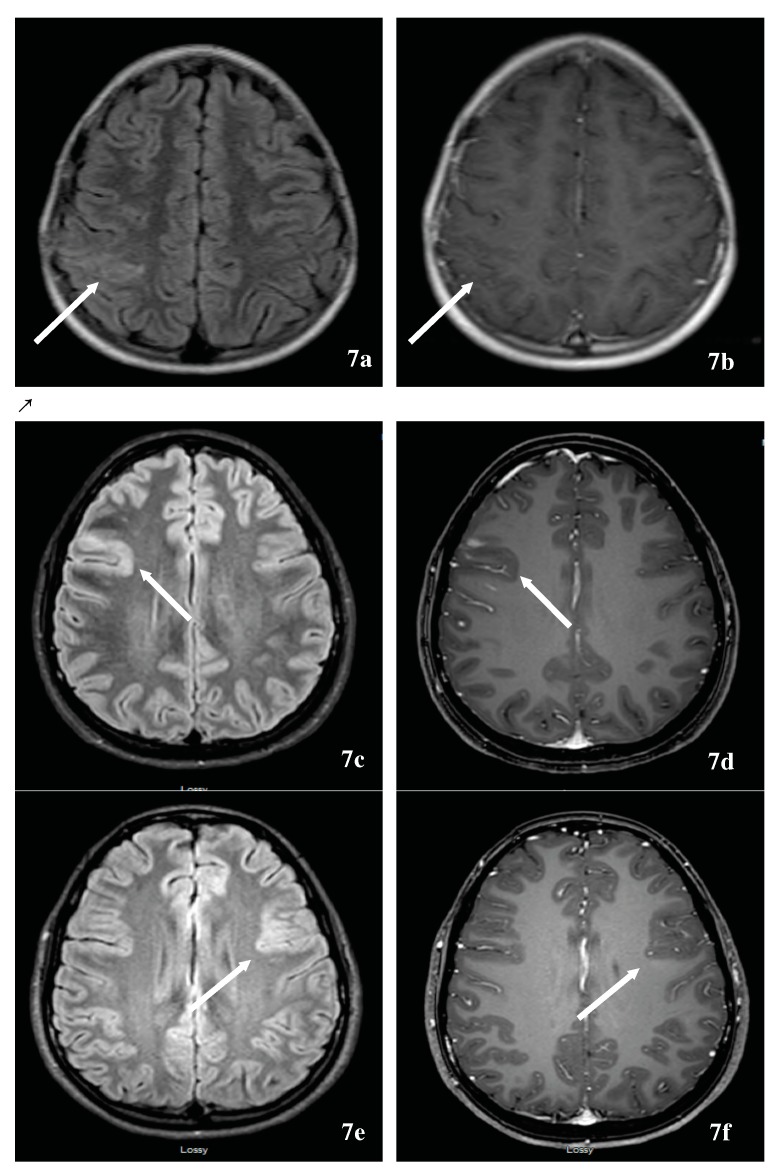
This is the case of a 21-year-old woman who presented with a history of bilateral optic neuritis at the age of 10. She subsequently had recurrent encephalitis with cortical swelling and seizures at the age of 13, 17 and 19. Axial FLAIR shows a right parietal cortical T2 hyperintensity with swelling (7a) associated with minimal T1 hypointensity without contrast enhancement (7b). Recurrent encephalitis was associated with a new right frontal cortical T2 hyperintensity with swelling (7c) associated with minimal T1 hypointensity with contrast enhancement. (7d). A third episode demonstrated a new left frontal cortical T2 hyperintensity with swelling (7e) associated with minimal T1 hypointensity with contrast enhancement (7f). Serum MOG-antibody was positive.
